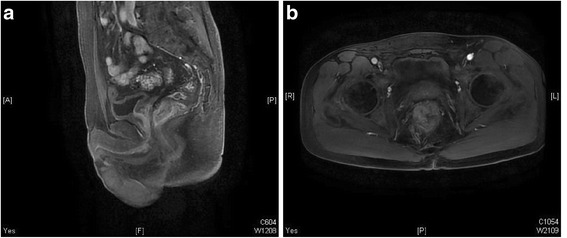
Primary lesion of case 1. The primary lesion was detected in the upper rectum on magnetic resonance imaging, which also revealed swollen pararectal lymph nodes. a Sagittal section; b: transverse section
